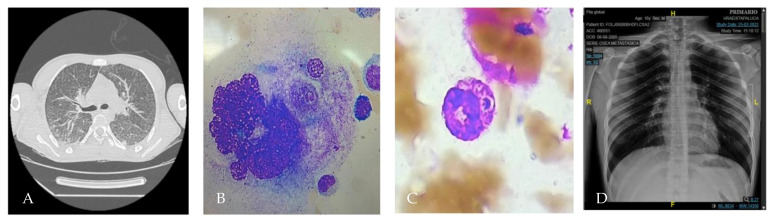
Timeline of the case, highlighting the diagnoses and treatments of the HIV-positive pediatric patient in descending chronological order (the diagnosis is highlighted in colored typography, and the corresponding treatment is indicated using the same color). (A) Tomography of the patient upon hospital admission showing the presence of characteristic histoplasmosis nodules; (B) the identification of yeasts with the typical characteristics of *Histoplasma* sp. In the bone marrow aspirate (100×); (C) structures of *Ehrlichia* sp. (100×); (D) thoracic X-ray after nine months of treatment, showing a decrease in the pulmonary radiopacity identified upon admission.
